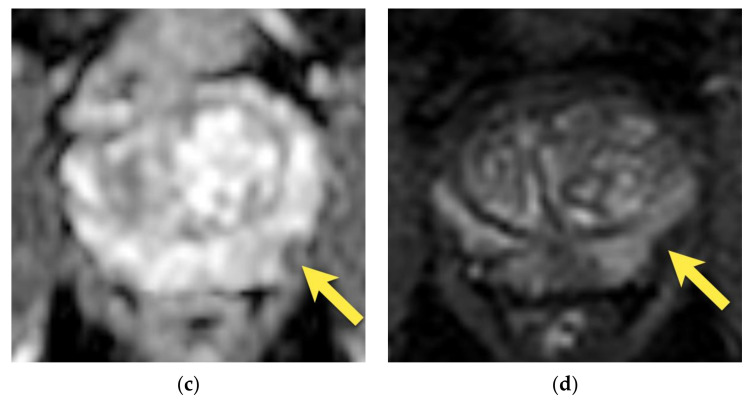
Periprostatic venous plexus in the left postero-lateral side of the prostate, presenting on axial images as an oval structure with: (a) intermediate to low signal intensity on T2-w image on the left postero-lateral side of prostate, due to turbulent flow (arrow); moderate diffusion restriction; (b) increased signal on DWI b 2000 image (arrow); (c) decreased signal on ADC map (arrow); (d) but with no early focal enhancement (arrow).
